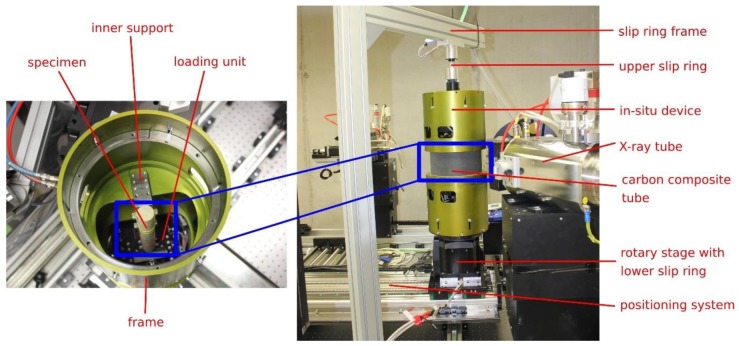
The in-situ loading device attached to the rotary stage of the X-ray scanner.
